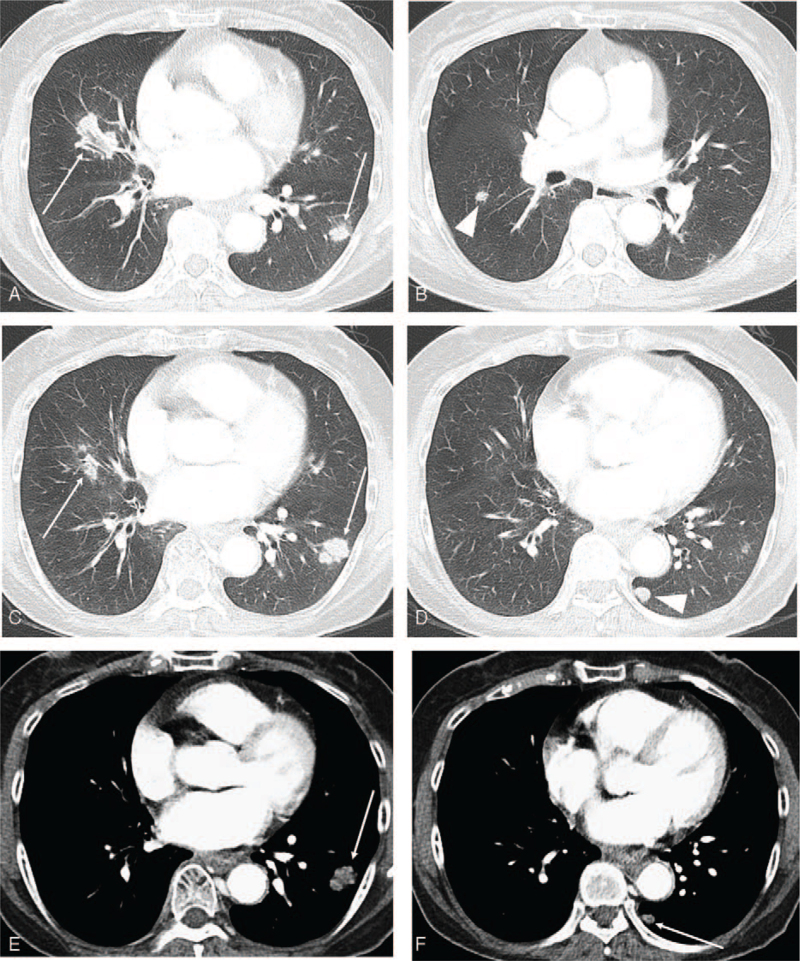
A follow-up contrast-enhanced chest CT performed at our hospital at 1 month after the initial chest CT scanning at the referring center. (A—D) On lung window setting, enlargement of pulmonary nodules (arrows) and newly appeared nodules in the right middle lobe and the left lower lobe are seen (arrowheads). Peribronchovascular distribution of the pulmonary nodules is well demonstrated (arrows). (E,F) On mediastinal window setting, nodules in left lower lobe show areas of low attenuation (arrows) representing necrosis.
